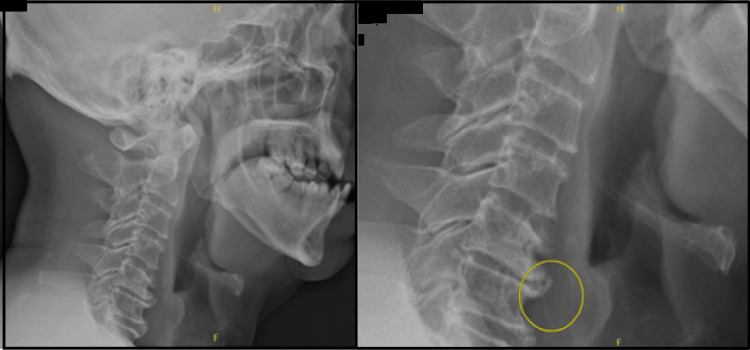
Cervical x-ray of the patient in lateral view showing foreign body in the marked area
